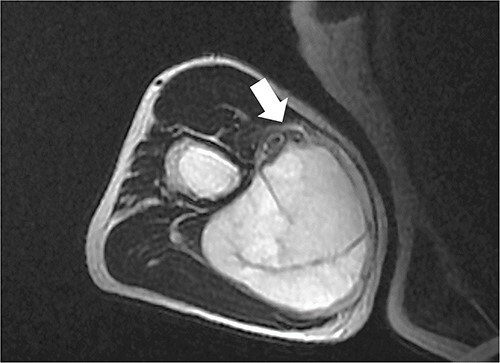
T2-weighted axial magnetic resonance imaging of the right upper arm. High-intensity mass can be seen between the biceps and triceps. The white arrow indicates the neurovascular bundle.
